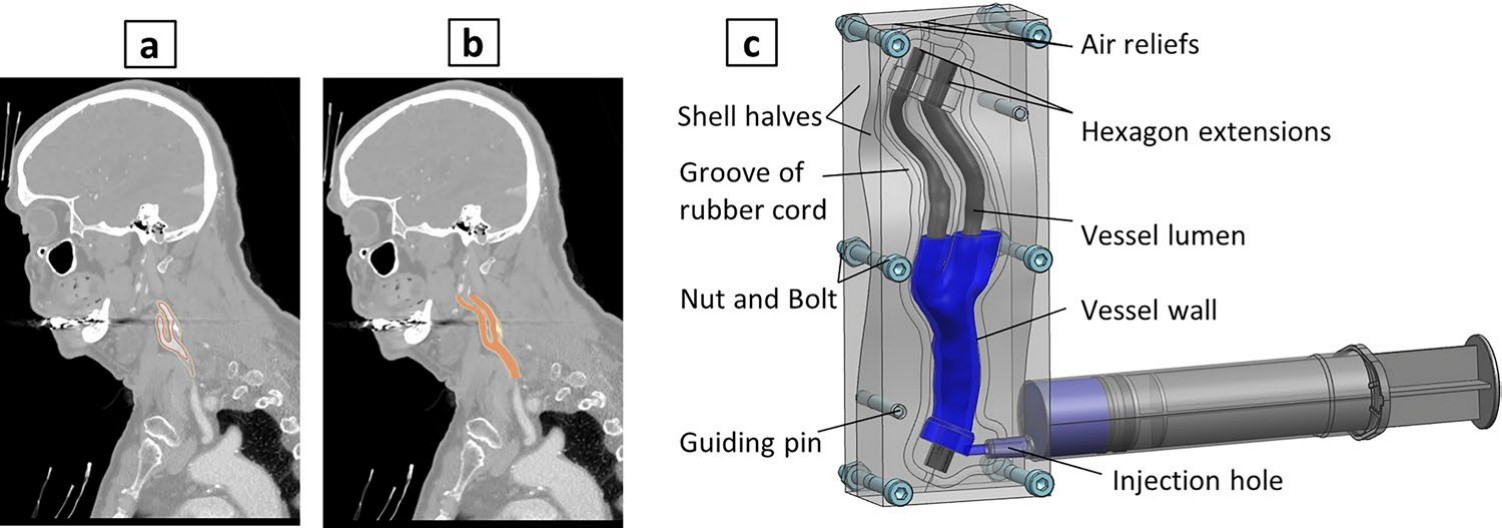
2D image cut (a) and projection (b) of the reconstructed carotid artery bifurcation on CTA sagittal slice of patient. 3D model depicting material injection into the core–shell mold of the carotid vessel (c).
